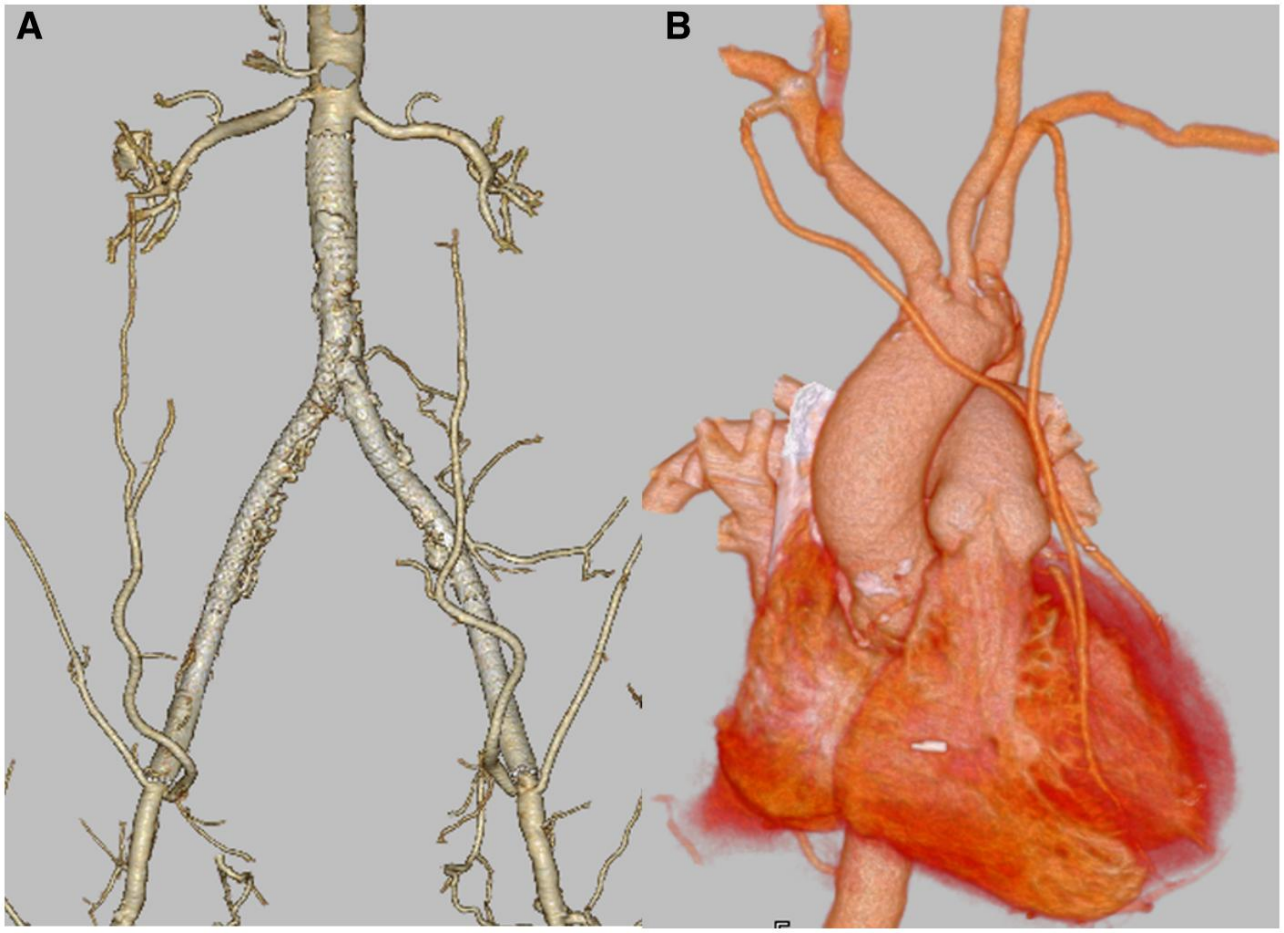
(A and B) Postoperative 3D computed tomography showed good blood flow of the aortoiliac artery and patent bypass
